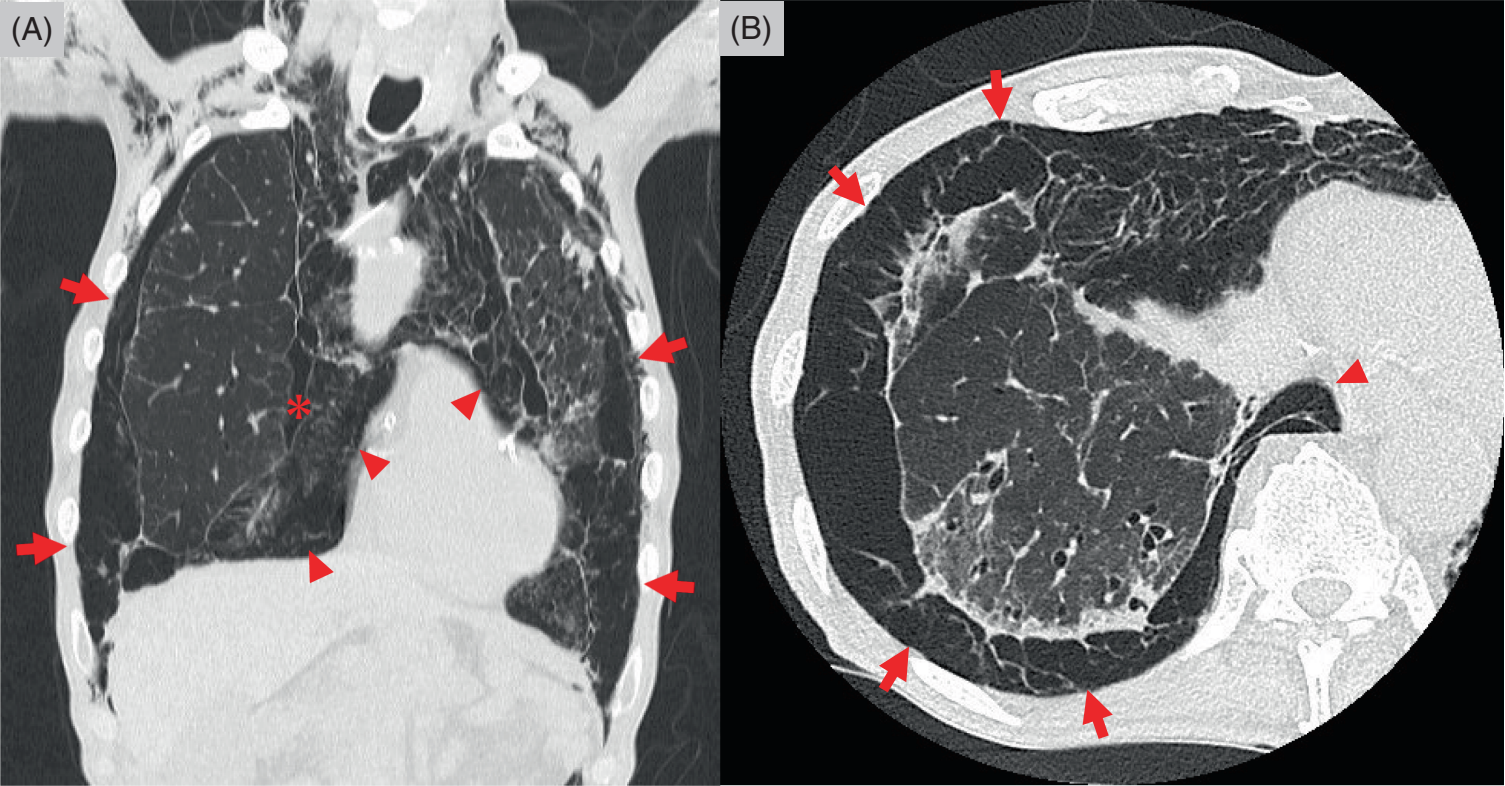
Chest computed tomography scan in coronal (A) and axial (B) views shows a space of fragile connective tissue and mediastinal emphysema surrounding the lungs: extrapleural air.
